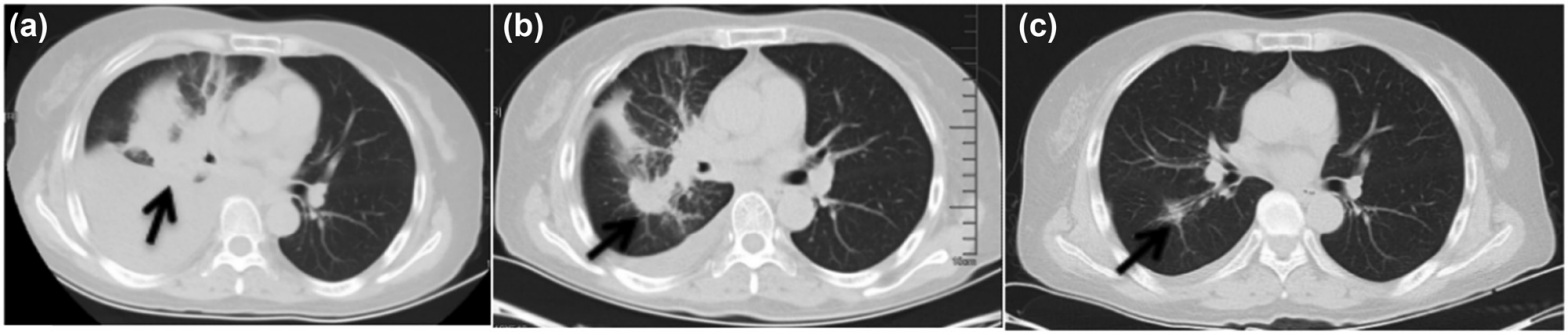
Chest CT. The arrow points to the tumor site. (a) At initial diagnosis, (b) after closed drainage of the right chest, and (c) after targeted therapy of gefitinib.
